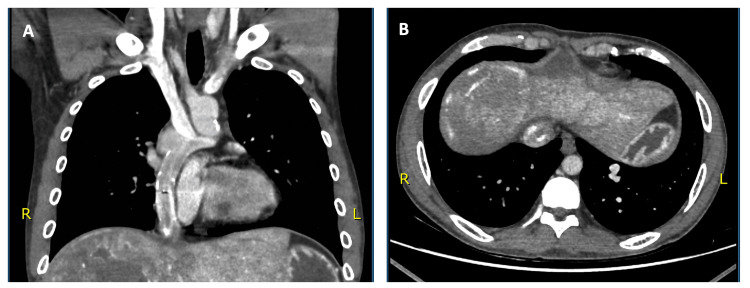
contrast-enhanced CT of the chest A: Coronal image showing anomalous systemic and pulmonary venous return. B: Axial image showing a vascular malformation measuring 3 × 1.4 cm noted at the base of the left lung.
